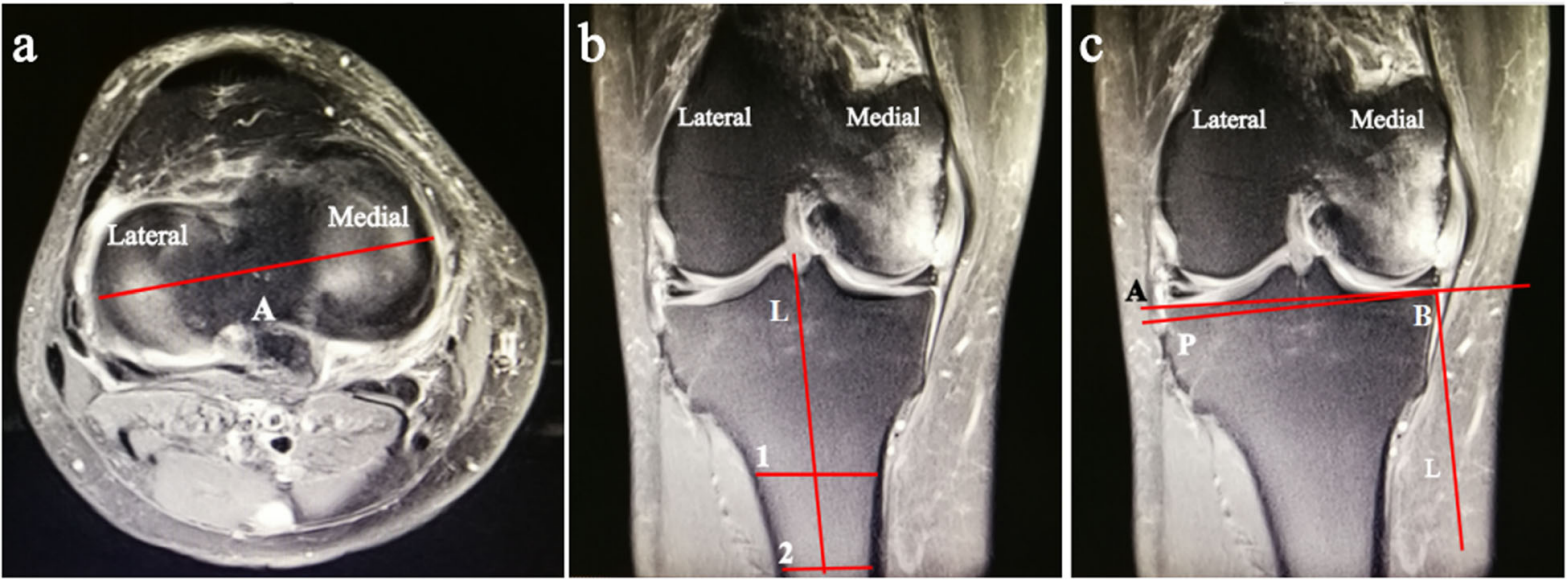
Magnetic resonance images depicting the method used to obtain the coronal slopes. a This shows the transverse plane passing through the tibiofemoral joint and showing the dorsal aspect of the tibial plateau. Line A shows the location of the coronal plane that passed closest to the centroid of the tibial plateau. b The longitudinal axis (line L) of the tibia was obtained by determining the midpoint of the medial-to-lateral width of the tibia at two points located as distally in the image as possible (locations 1 and 2). c The coronal tibial slope was the angle between a line joining the peak points on the medial and lateral aspects of the plateau (points A and B) and line P perpendicular to line L
